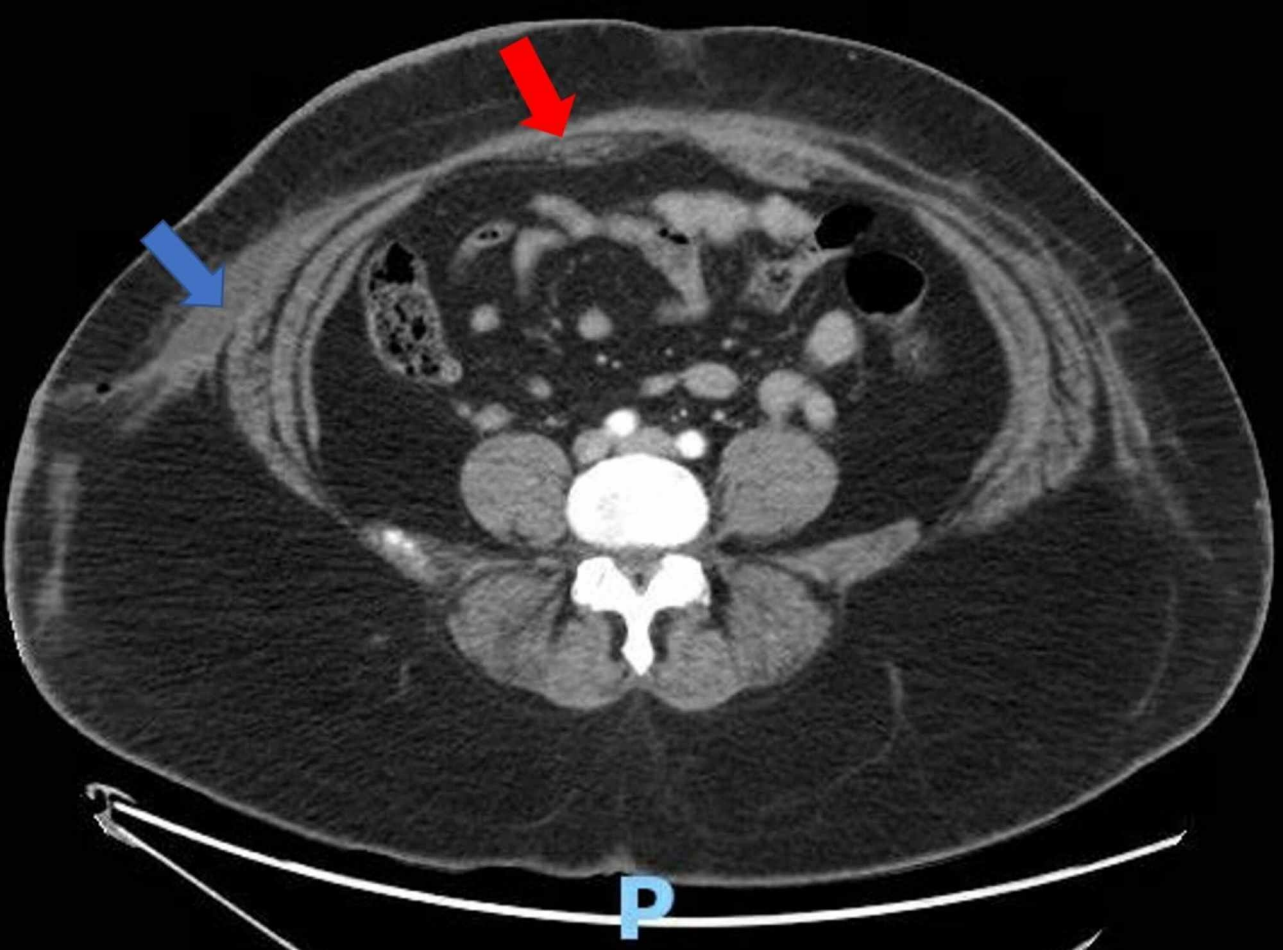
CT scan: transverse view of the abdominal cavity. Again, the mixed attenuated fluid collection in the right anterolateral abdominal wall (blue arrow) is seen. The midline fluid collection (red arrow) is better visualized. The letter P denotes the posterior orientation of the patient.
